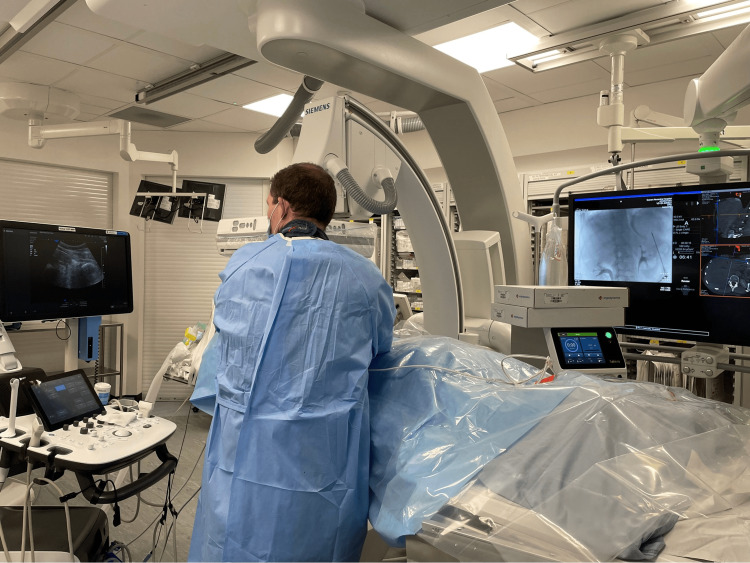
Interventional radiology suite arrangement The anaesthetist stands at the head of the patient, the radiologist is on the right of the patient, the ultrasound machine is in front of the operator, and the thermal ablation machine and screens are opposite the operator. The nurse stands on the left of the patient, and the radiographer stands at the foot of the patient.
